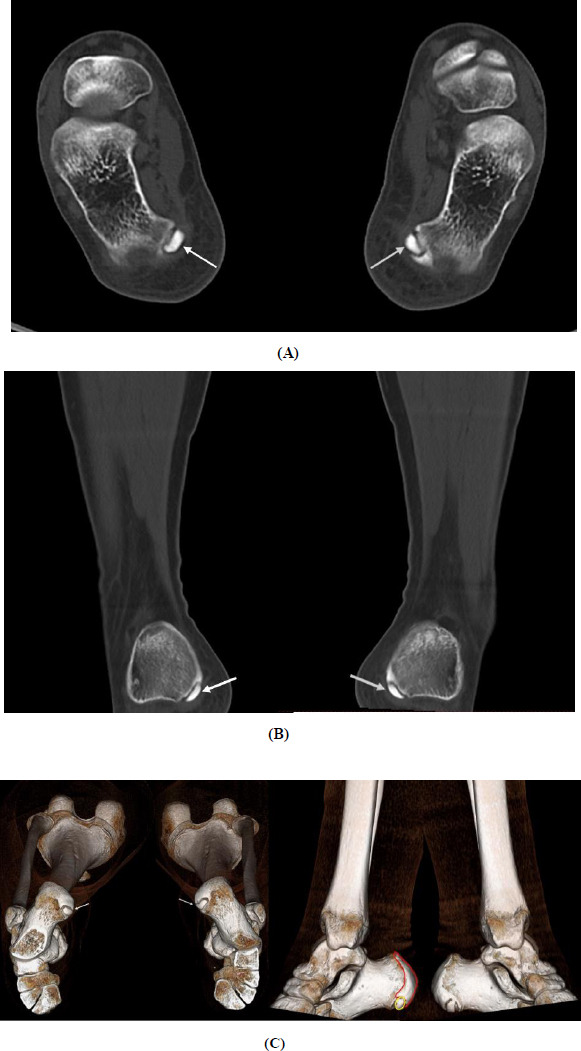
3-dimensional non-contrast computed tomography (CT) scan of a 12-year-old female. Both axial (A), coronal (B) reformatted images, and VR images (C) show bilateral unfused medial calcaneal apophyses (white arrows and yellow circle) with well-defined corticated margins. The ossicles measured 8.8 mm and 9.4 mm in maximal diameter on the left and right sides, respectively. (Note. Red circle: fused calcaneal apophysis)
